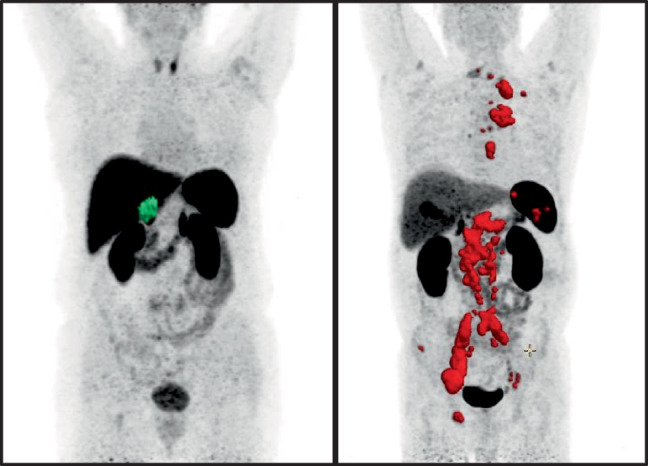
[^18^F]SiTATE-PET/CT in two PPGL patients with limited (left) and metastatic disease (right)
